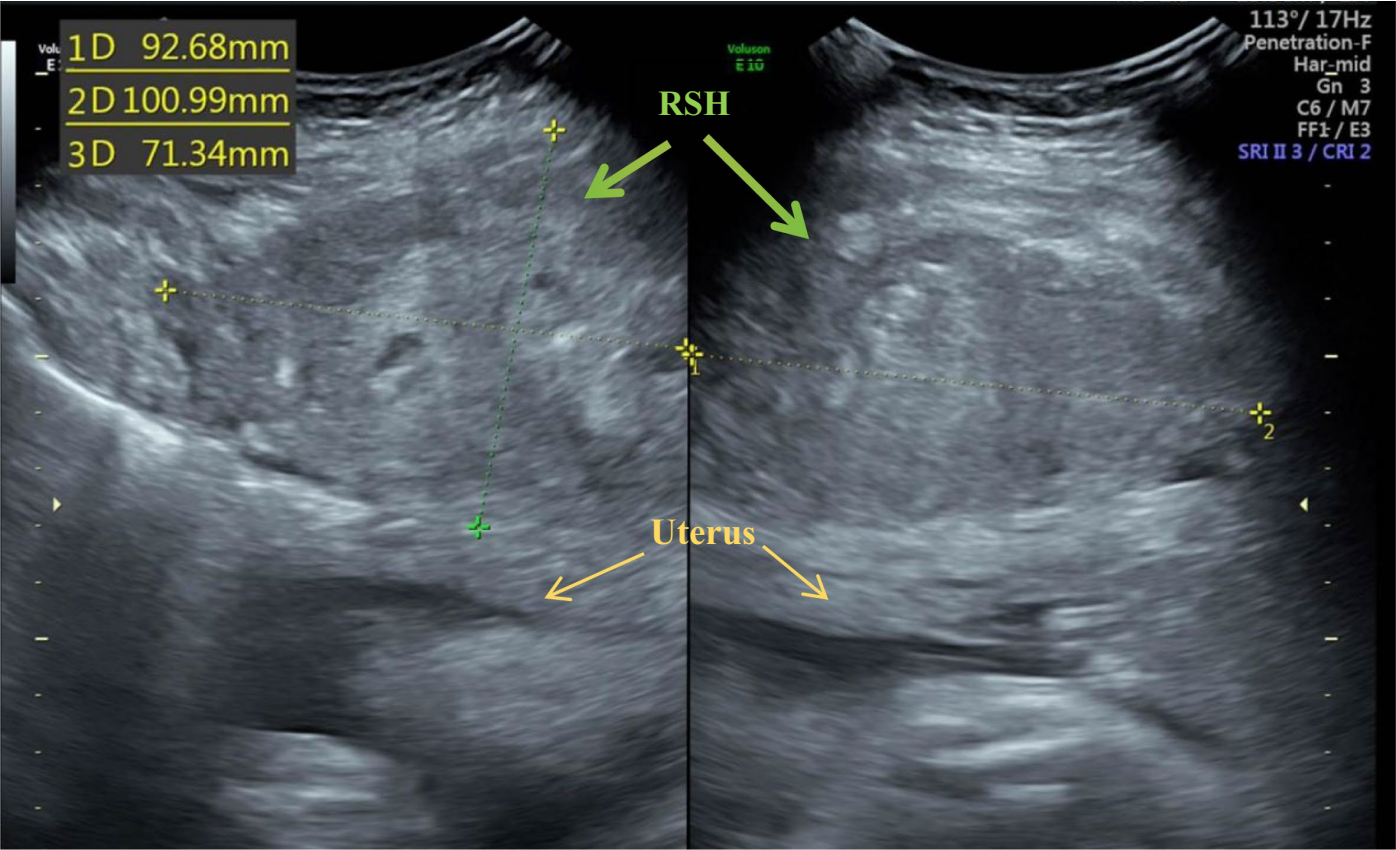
Ultrasonographic image shows the rectus sheath hematoma (RSH, green arrow) and the uterus (yellow arrow).
